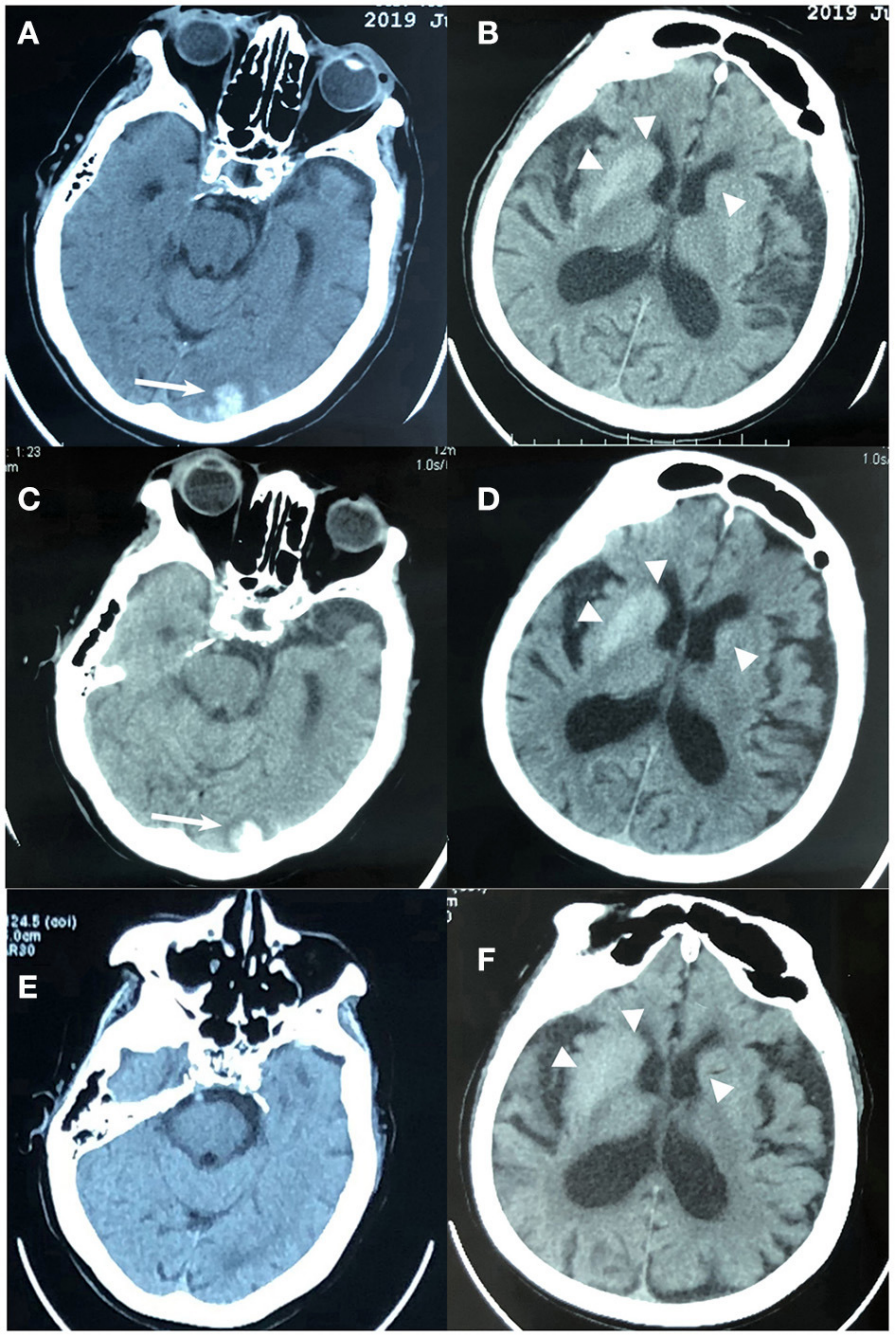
Brain computed tomography (CT) imaging of an 88-year-old woman with non-ketotic hyperglycemia chorea-ballism and intracerebral hemorrhage. (A,B) At admission, CT showed hyperdense lesions in the left occipital lobe (white arrow), right caudate nucleus and putamen (triangle), and left caudate (triangle). (C,D) Eight hours later after admission, CT showed mild hematoma enlargement, slightly increased edema around the occipital lobe lesion (white arrow), and no significant changes in the bilateral striatum regions (triangle); (E,F) 15 days before admission, CT showed hyperdensity in bilateral striatum regions (triangle) without edema and occipital lobe lesion.
